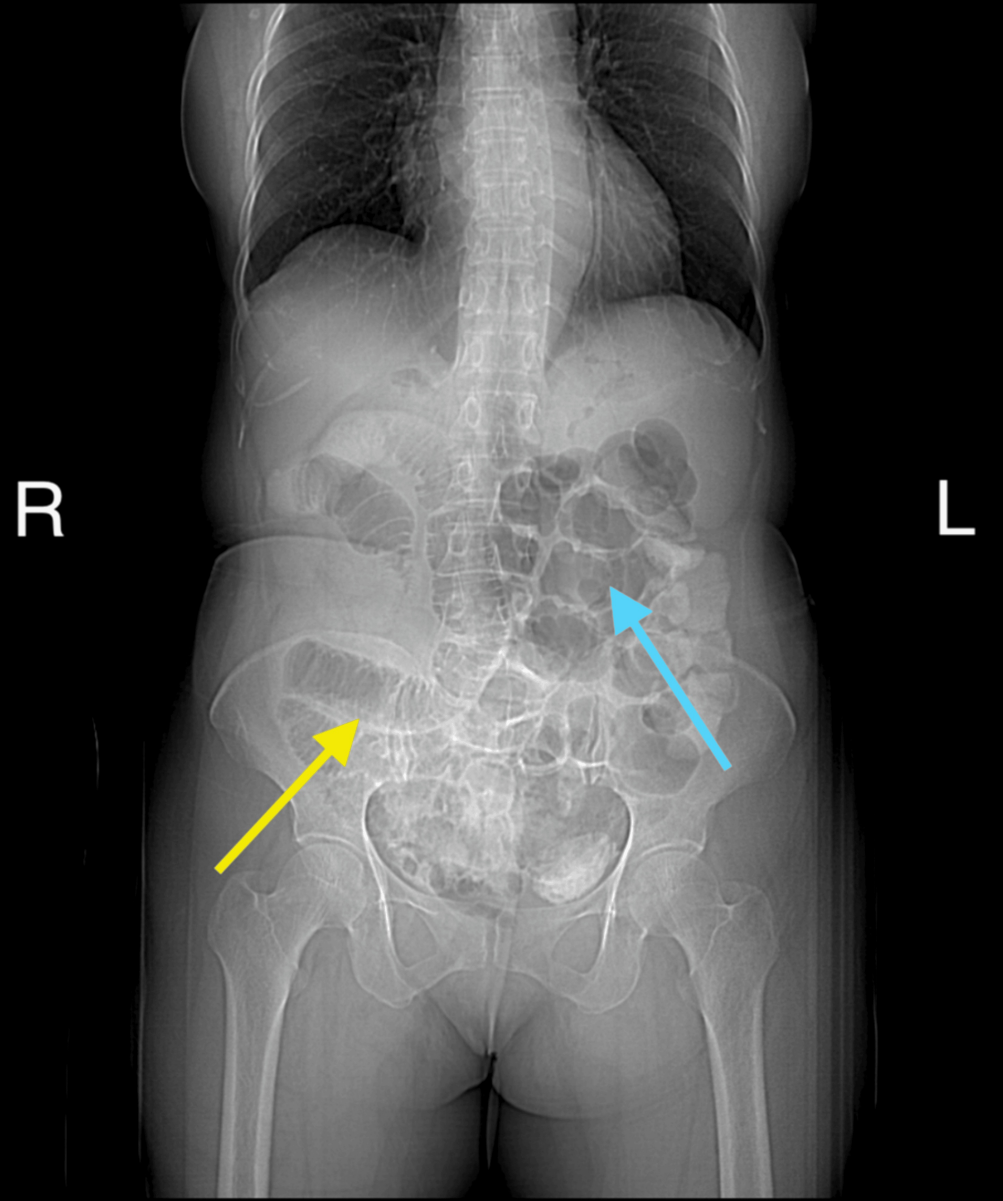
The AP view of a contrast-enhanced CT indicating the small bowel on the right side (yellow arrow) and the large bowel on the left side (blue arrow) of the abdomen AP: anteroposterior
